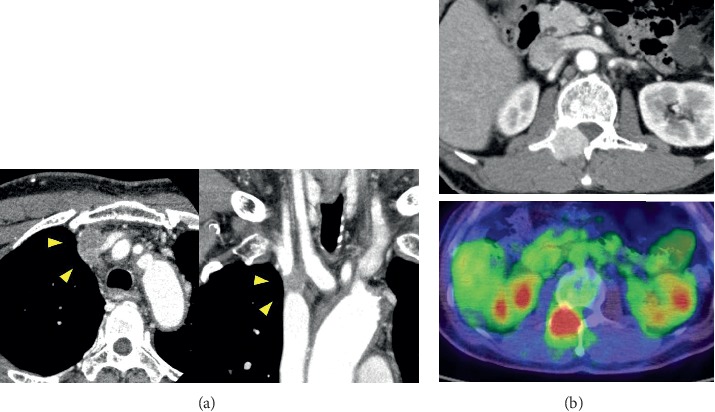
CT and PET-CT findings. (a) Computed tomography showing a 27 mm lump on the right side of the upper mediastinum that is invading the superior vena cava. (b) PET-CT showing metastasis of the lumbar vertebrae.
